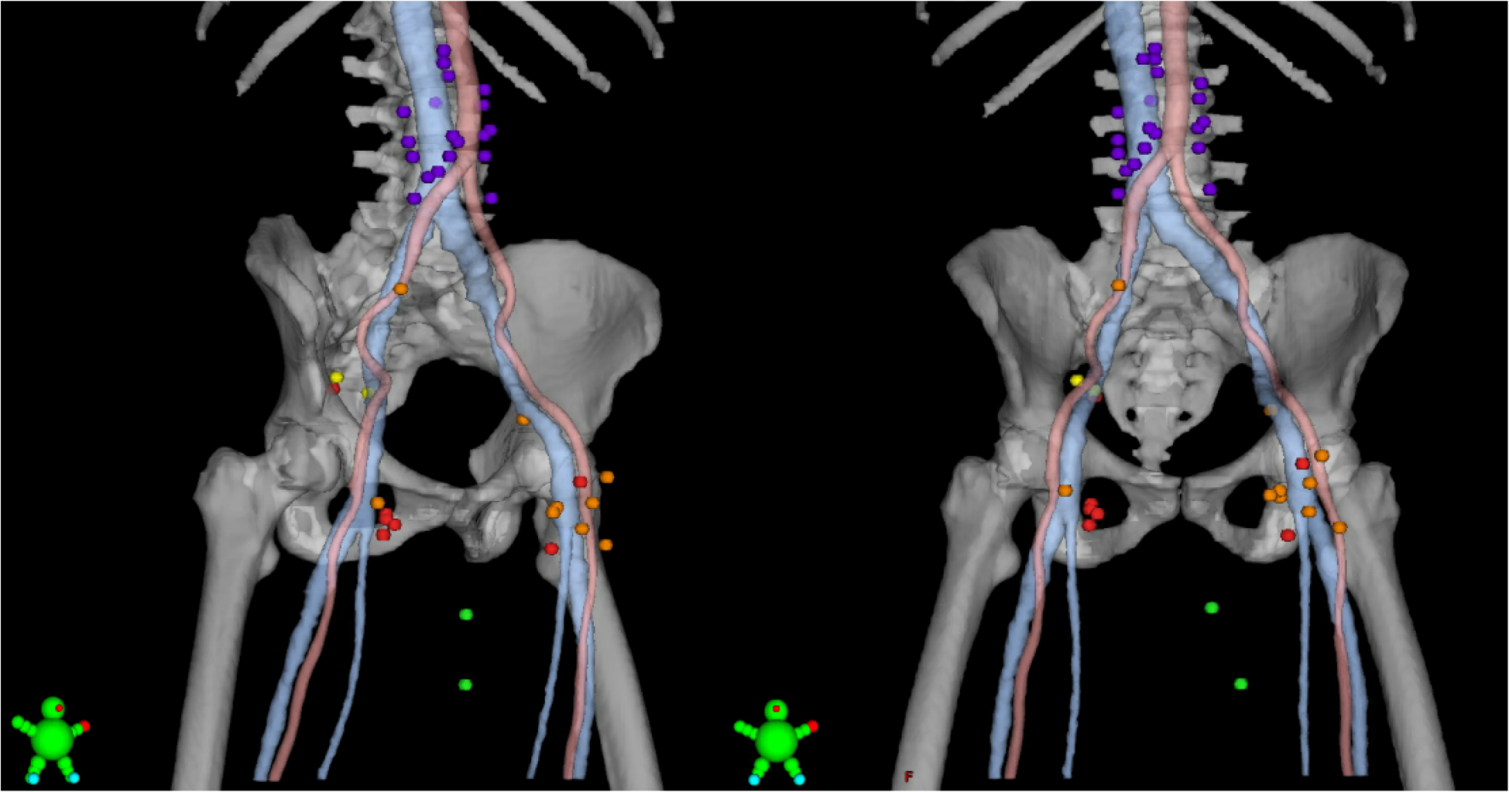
Regional, ano-inguinal lymphatic drainage (AILD), and common iliac/para-aortic (CI/PA) recurrences in 17 patients. Recurrence in GTVN (red; 7 patients, 7 lymph nodes); within elective CTV (orange; 7 patients, 10 lymph nodes); outside elective CTV but within pelvis or inguinal (yellow; 1 patient, 2 lymph nodes); AILD (green; 2 patients, 2 metastases); CI/PA (purple; 7 patients, 20 lymph nodes). Blue, inferior vena cava, external iliac, femoral, and great saphenous vein. Center of lymph node mapped with a 9 mm sphere in a standard anatomy reference CT
